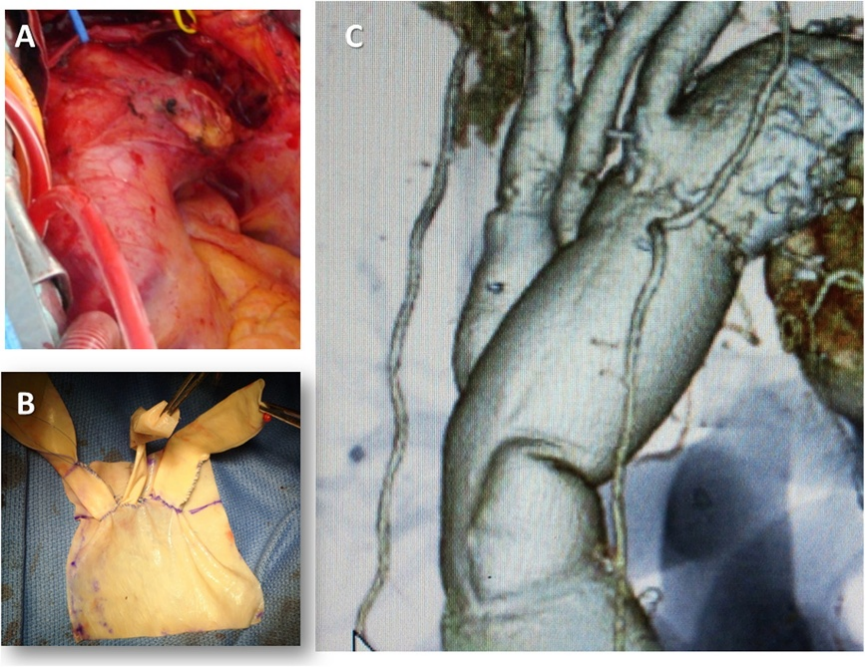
a The aneurysm was located in the proximal aortic arch (Case 2). b Three-branched pericardial sheet. c Postoperative 3-D computed tomography revealed no stenosis or dilatation of the xenopericardial roll graft branches
